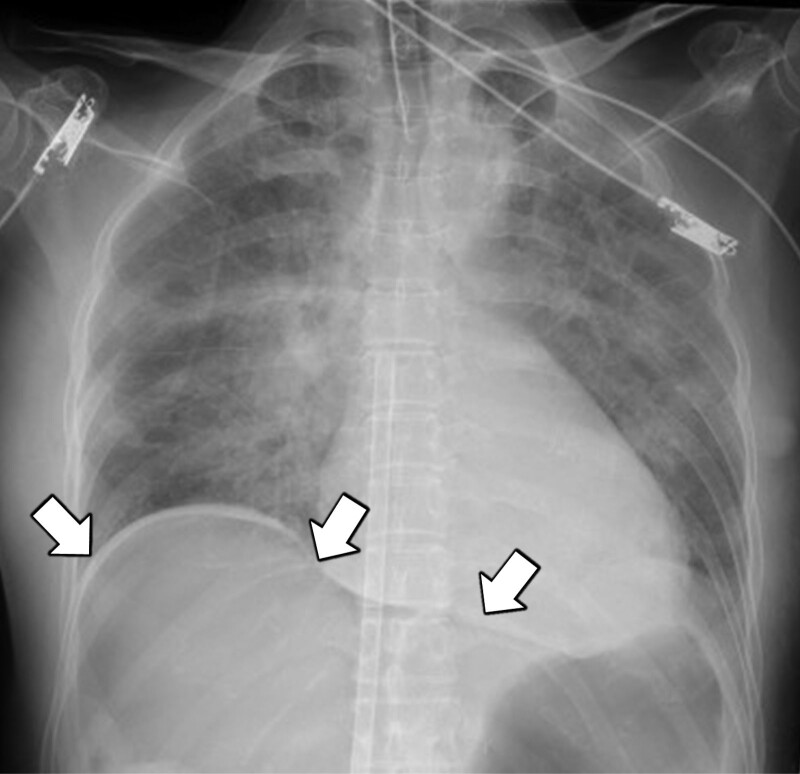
Chest radiograph obtained after VA-ECMO induction showing diffuse infiltrative shadows suggestive of acute respiratory distress syndrome and free air in the abdominal cavity (white arrows). VA-ECMO = venoarterial extracorporeal membrane oxygenation.
